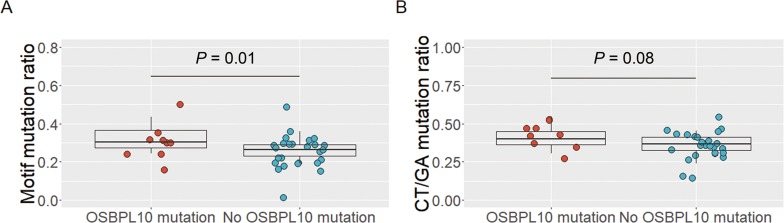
Analysis of *OSBPL10* mutations and somatic hypermutation target motifs (A) Proportion of RGYW/WRCY motif mutations to all somatic mutations. (B) Proportion of CT/GA mutations to all somatic mutations.
